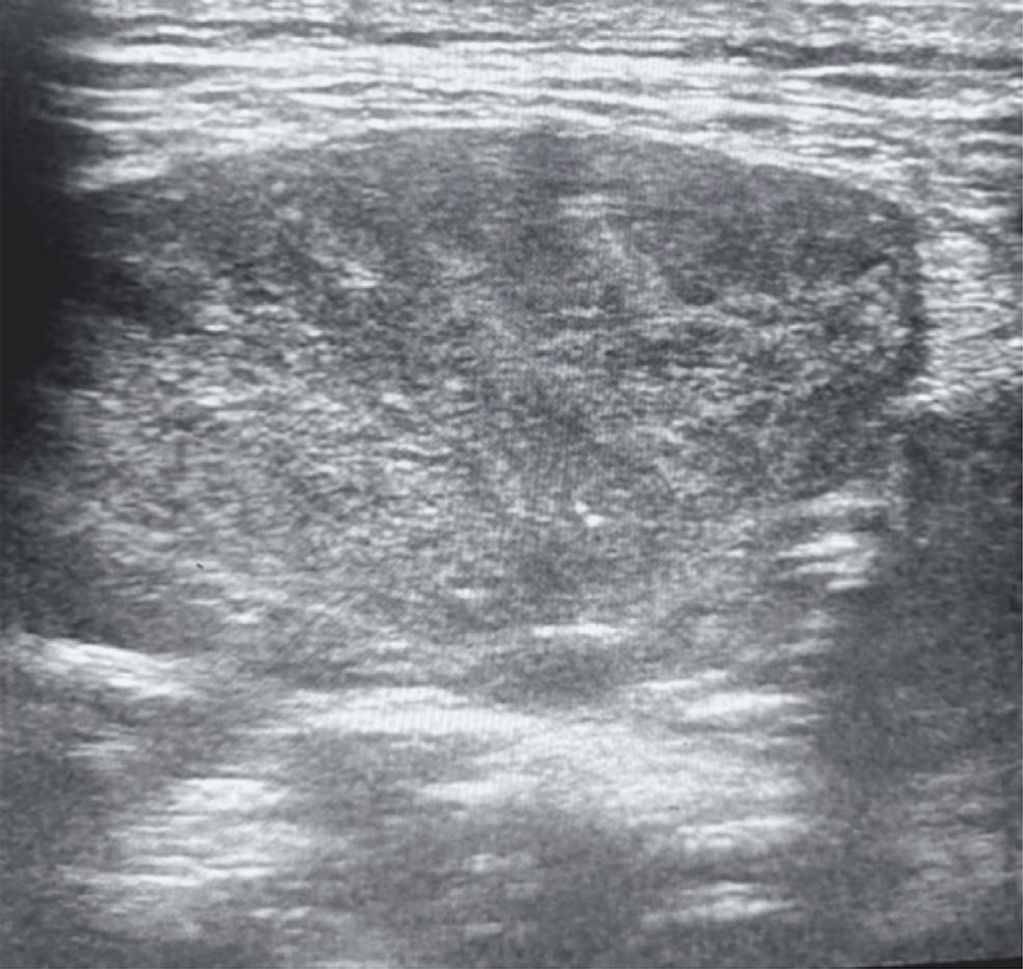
Ultrasound grey scale B mode-showing large mass lesion in the sacral region which appears heterogeneously hypoechoic with few areas of calcifications within.
